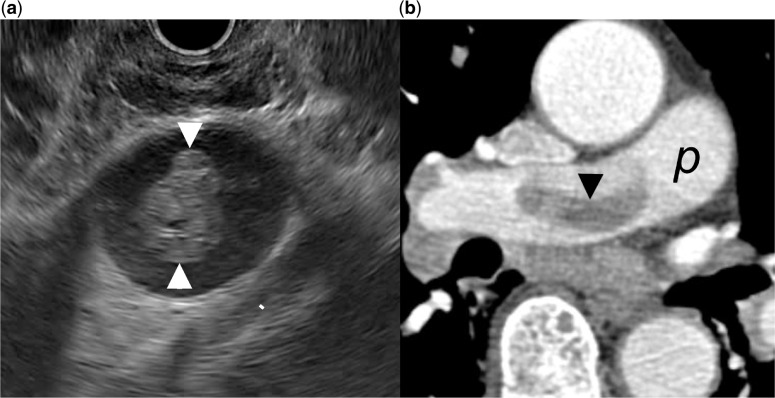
(a) Endoscopic ultrasound view of an intraluminal thrombus (arrowheads) of the main pulmonary artery. The endoscope is advanced at the mid-oesophagus and orientated towards the anterior wall of the oesophagus. (b) Computed tomography scan showed an extension of the thrombus to the right pulmonary artery (p).
